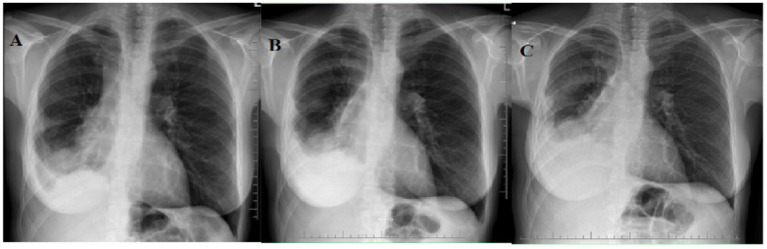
Patient No. 2’s chest X-ray examinations. (A) PA chest X-ray image at the time of treatment initiation shows a collection of fluid in the right pleural cavity; irregular interstitial thickening in the lower right lung is also visible. (B) PA chest X-ray image after 1 year of sirolimus treatment shows stabilization of the fluid collection in the right pleural cavity. (C) PA chest X-ray image after 5 years of sirolimus treatment shows a slight increase in the amount of fluid in the right pleural cavity.
